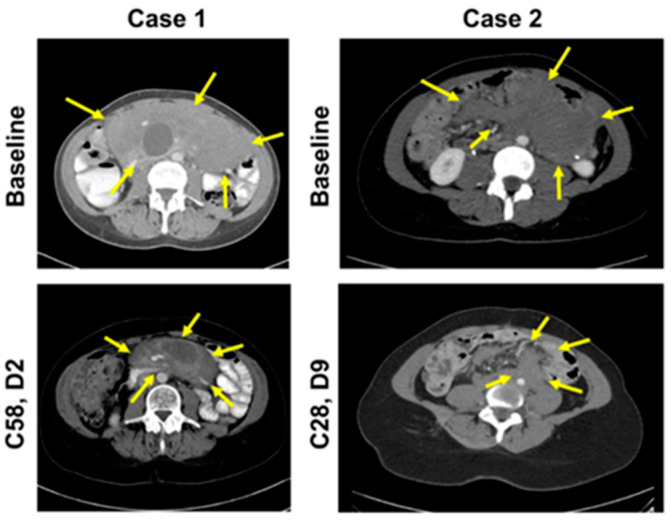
CT scans of target lesions at baseline and after achieving PR in both patients. Abbreviations: C, cycle; CT, computed tomography; D, day; PR, partial response.
